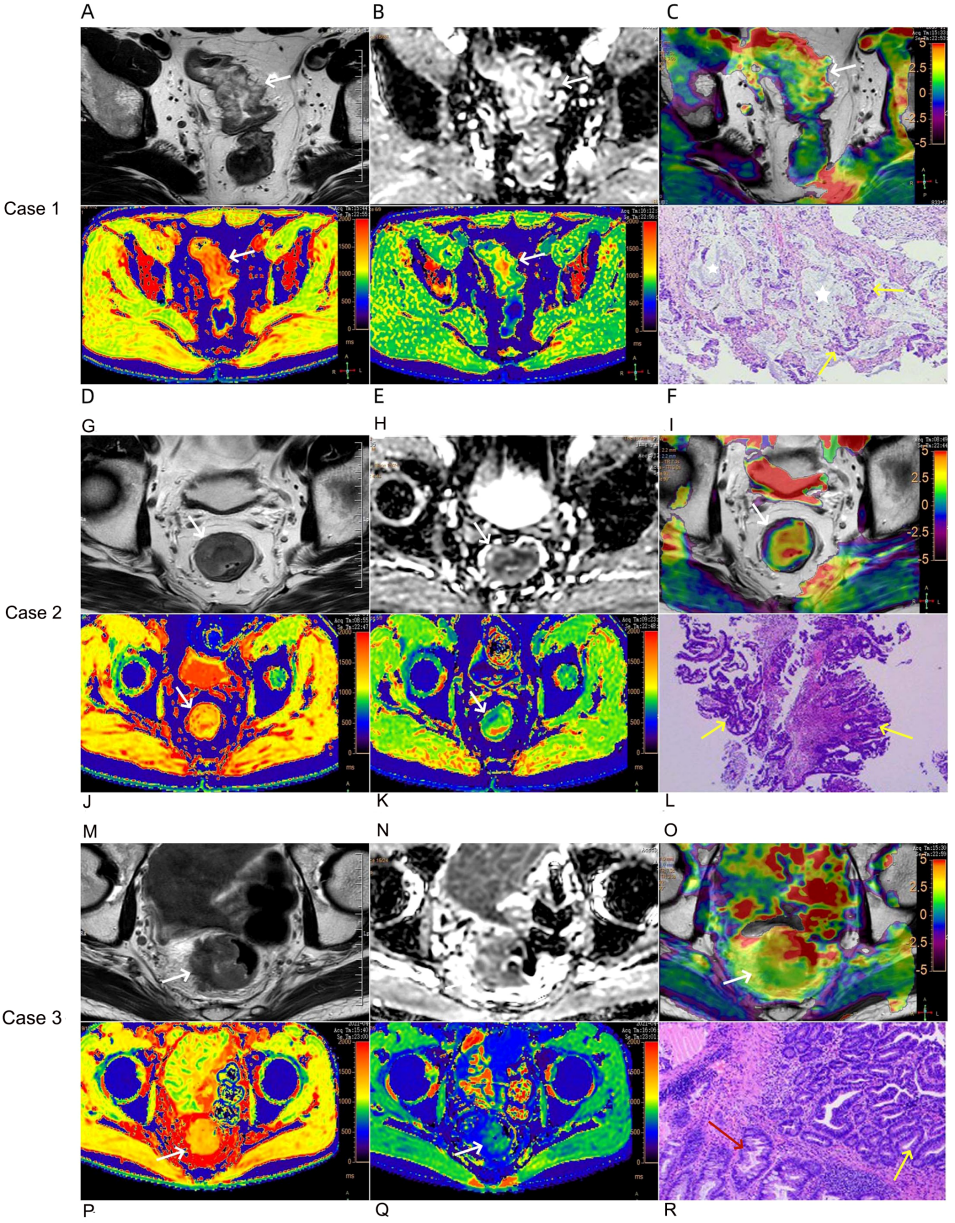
Case 1: A 76-year-old female with mucinous adenocarcinoma (A–F). Oblique axial T2-weighted image showed the rectal wall thickens with a high-intensity signal (A). The lesion showed a slightly high-intensity signal on the ADC map, the ADC value was 1.15×10^-3^ mm^2^/s (B). APT-T2 merged image showed the mass with a mean APT SI of 3.87% (C). At native T1 pseudo-color image, the lesions were mainly red, and the relaxation time of T1 was 1660 ms (D). At post-contrast T1 pseudo-color image, the lesions were mainly green, and the relaxation time of T1 was 652 ms (E). Pathological sections showed mucous adenocarcinoma of the rectum (HE,×200) (F), tumor glandular epithelium lining around the mucinous pool, the yellow arrow represents the tumor glandular epithelium, and the white five pointed star area represents the mucinous pool. Case 2: A 52-year-old male with common adenocarcinoma,T2N0, EMVI-, PNI-, LVI- (G–L). Oblique axial T2-weighted image showed the mass with a slight high-intensity signal (G). The lesion showed a slightly low-intensity signal on the ADC map, the ADC value was 0.93×10^-3^ mm^2^/s (H). APT-T2 merged image showed the mass with a mean APT SI of 1.17% (I). At native T1 pseudo-color image, the lesions were mainly yellow, and the relaxation time of T1 was 1250 ms (J). At post-contrast T1 pseudo-color image, the lesions were mainly green, and the relaxation time of T1 was 819 ms (K). Pathological sections showed common adenocarcinoma of the rectum (HE,×200) (L), the yellow arrow represents the tumor glandular epithelium. Case 3: A 63-year-old male with common adenocarcinoma,T3N1, EMVI+, PNI-, LVI+ (M–R). Oblique axial T2-weighted image showed the mass with a slight high or iso-intensity signal (M). The lesion showed a low-intensity signal on the ADC map, the ADC value was 0.87×10^-3^ mm^2^/s (N). APT-T2 merged image showed the mass with a mean APT SI of 2.68% (O). At native T1 pseudo-color image, the lesions were mainly yellow, and the relaxation time of T1 was 1420 ms (P). At post-contrast T1 pseudo-color image, the lesions were mainly green, and the relaxation time of T1 was 717 ms (Q). Pathological sections showed common adenocarcinoma of the rectum (HE,×200) (R), the yellow arrow represents the tumor glandular epithelium and the red arrow represents normal epithelium.
